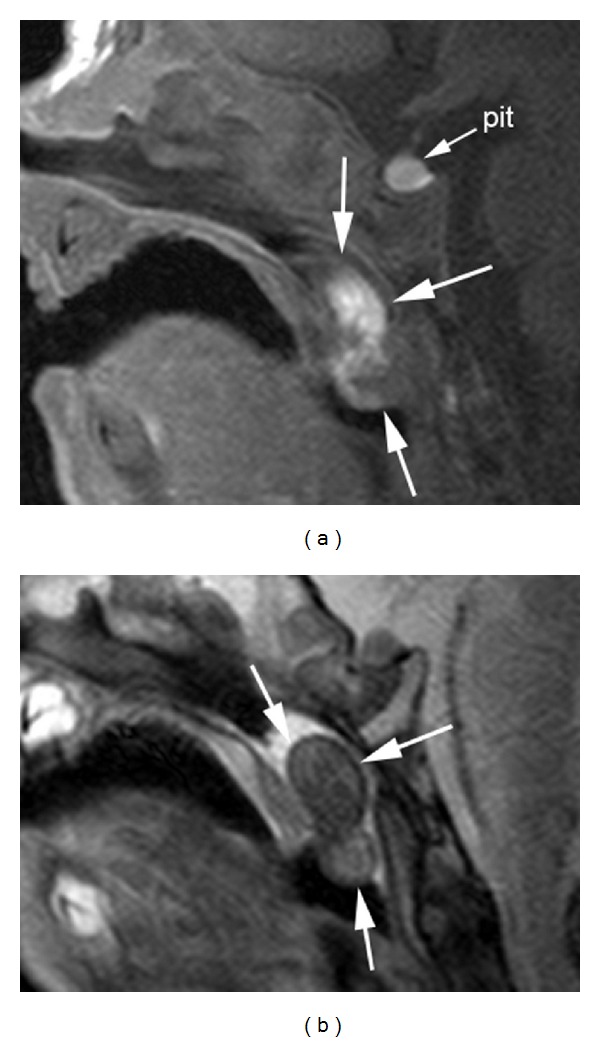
Sagittal short tau inversion recovery (STIR) (a) and sagittal T1-weighted (b) images of the nasopharynx show an oval, well-circumscribed mass (arrows) that is intimately related to the posterior aspect of the soft palate, but separate from it. The lesion is predominantly hyperintense on T1-weighted images and moderately and heterogeneously hyperintense on the T2-weighted images, features suggestive of fat content. Note the normal T1-weighted hyperintensity of the anterior pituitary (pit) in a newborn, presumably related to hypertrophy of the gland due to circulating maternal hormones.
